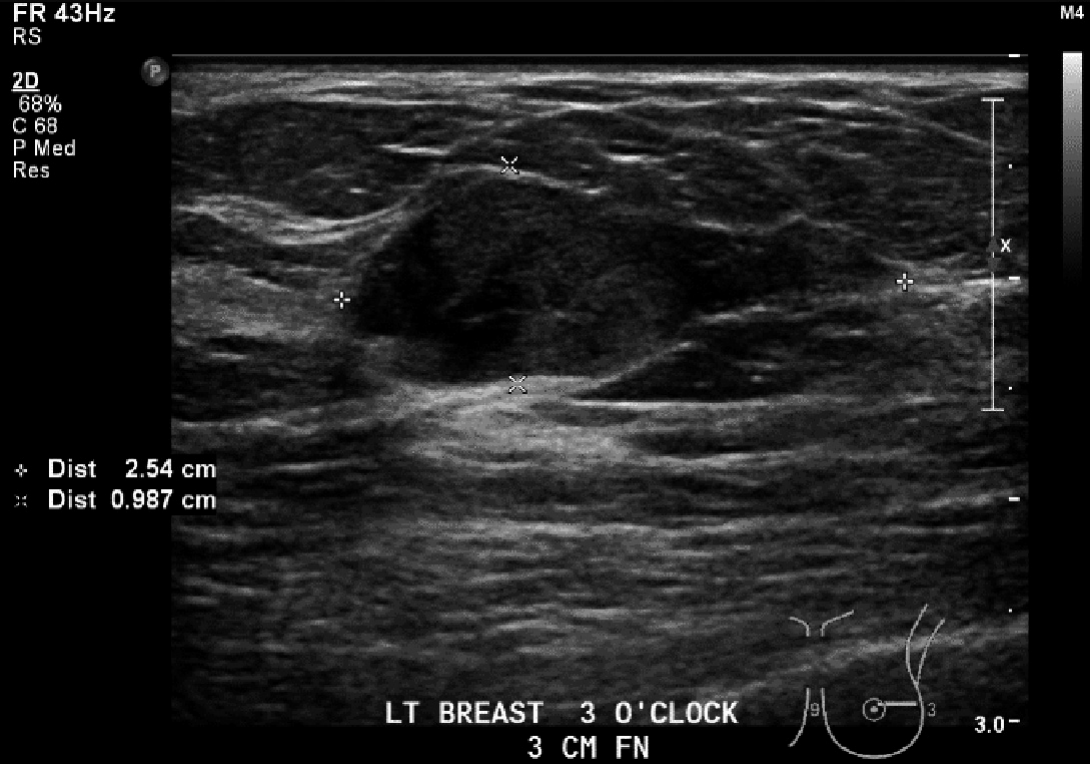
Lesion One: Ultrasound image showing a hypoechoic, well‐circumscribed mass with focus of internal calcification and small cystic component. Image courtesy of BreastScreen ACT.
